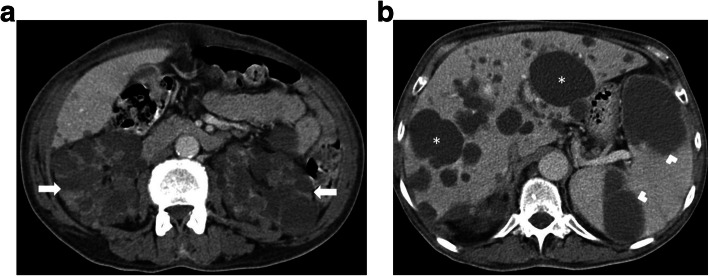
A 63-year-old male patient with known ADPKD. a Axial plane post-contrast CT image shows enlarged kidneys with innumerable cysts (arrows). b More cranial CT image demonstrates multiple hepatic (asterisks) and splenic cysts (arrowheads)
